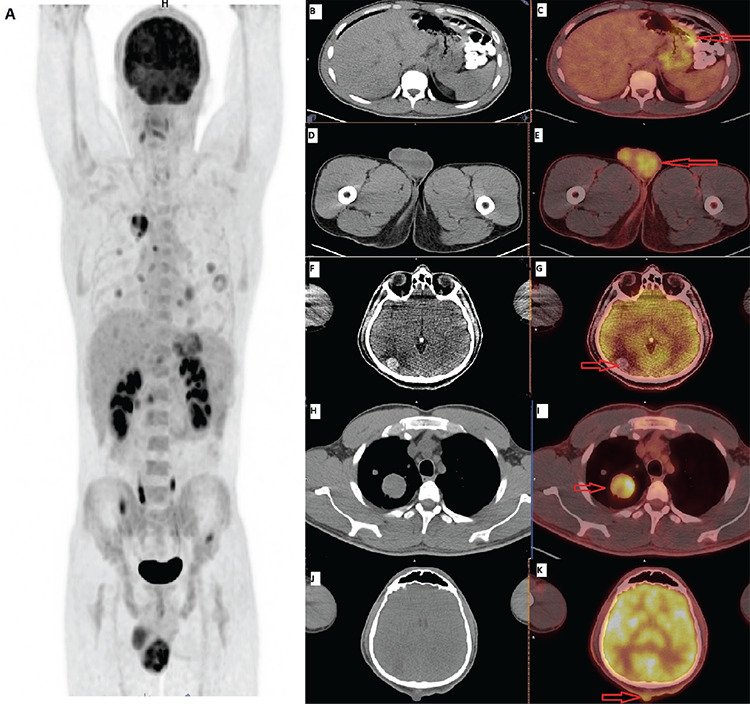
Testicular choriocarcinoma diagnosed with gastric metastases is extremely rare in the literature (1,2,3,4). A 27-year-old male presented with anemia and melena. Polypoid ulcerated lesion on the gastric greater curvature with active bleeding was detected using gastroscopy. The patient underwent ^18^fluorine-fluorodeoxyglucose (^18^F-FDG) positron emission tomography/computed tomography (PET/CT) for clinically suspected gastric cancer. Focal ^18^F-FDG uptake was found on the gastric greater curvature (A, B, C). Metastatic gastrointestinal involvement may be seen in approximately 5% of these cases (5,6). Hypermetabolic focus and asymmetric growth were also found in the left testicle (D, E), and multiple metastatic disease that involves the brain (F, G), lungs (H, I), skin (J, K), liver, lymph node, and bone was detected on PET/CT. Based on PET/CT, all metastases were thought to arise from the testicles. As in this case, in addition to the contribution of PET/CT in diagnosis of testicular cancer, it is very important imaging technique in clinical practice in staging and detection of recurrence (7).
